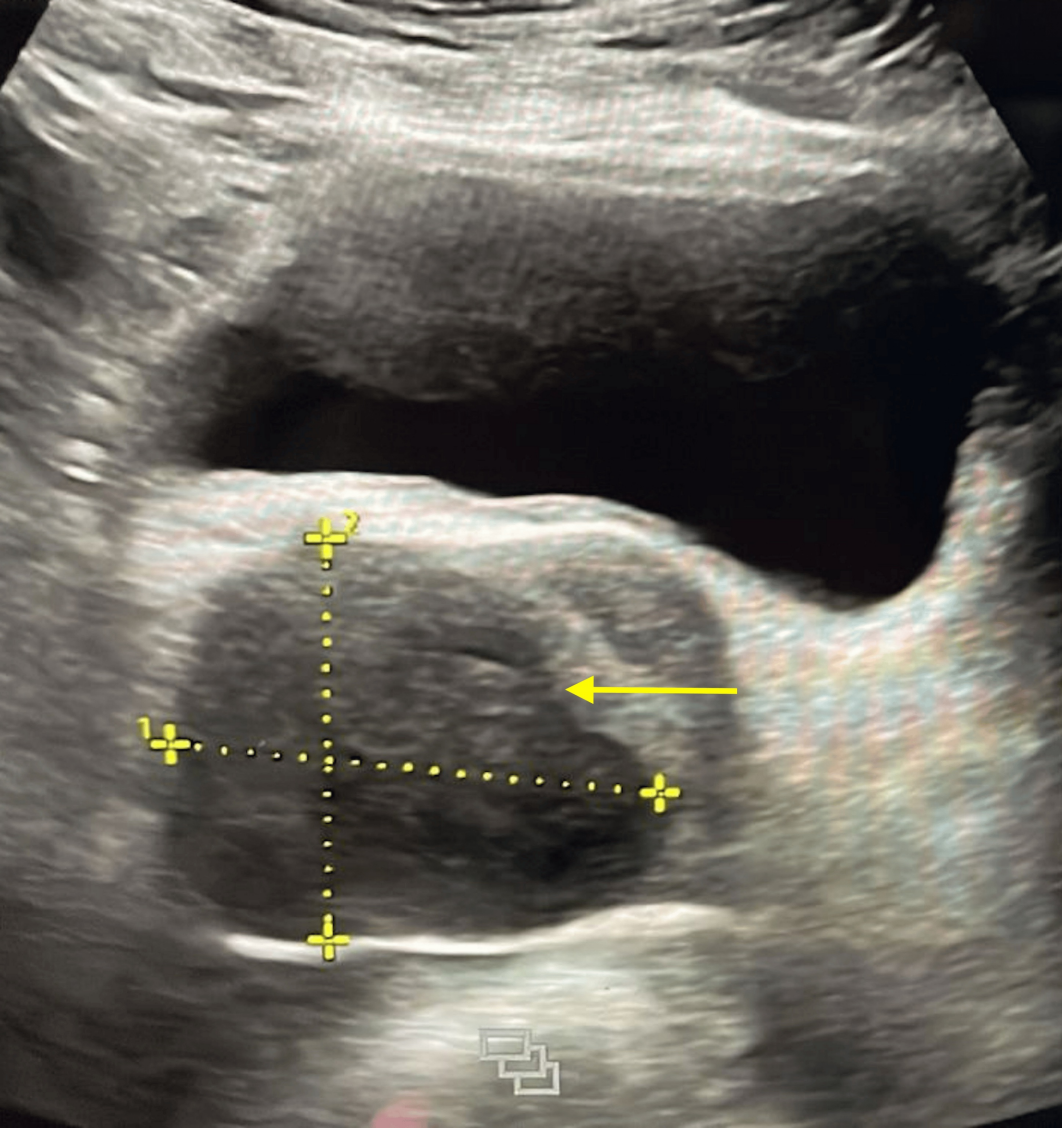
Transabdominal ultrasonography (USG) pointing with yellow arrow at a large well-defined heterogeneous hypoechoic lesion in the anterior wall of the lower uterine segment
